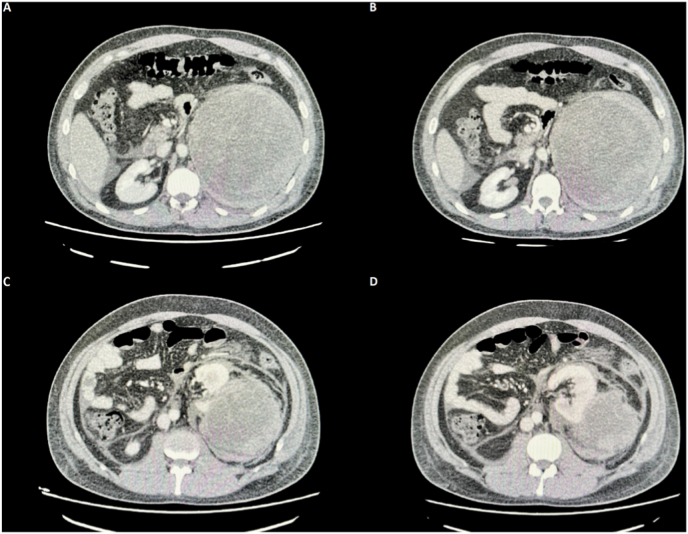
Abdominopelvic triphasic CT scan at different axial sections. Showing the tumor at multiple levels, denoting its maximum diameter, the edging towards the midline, and its proximity to the left kidney.
